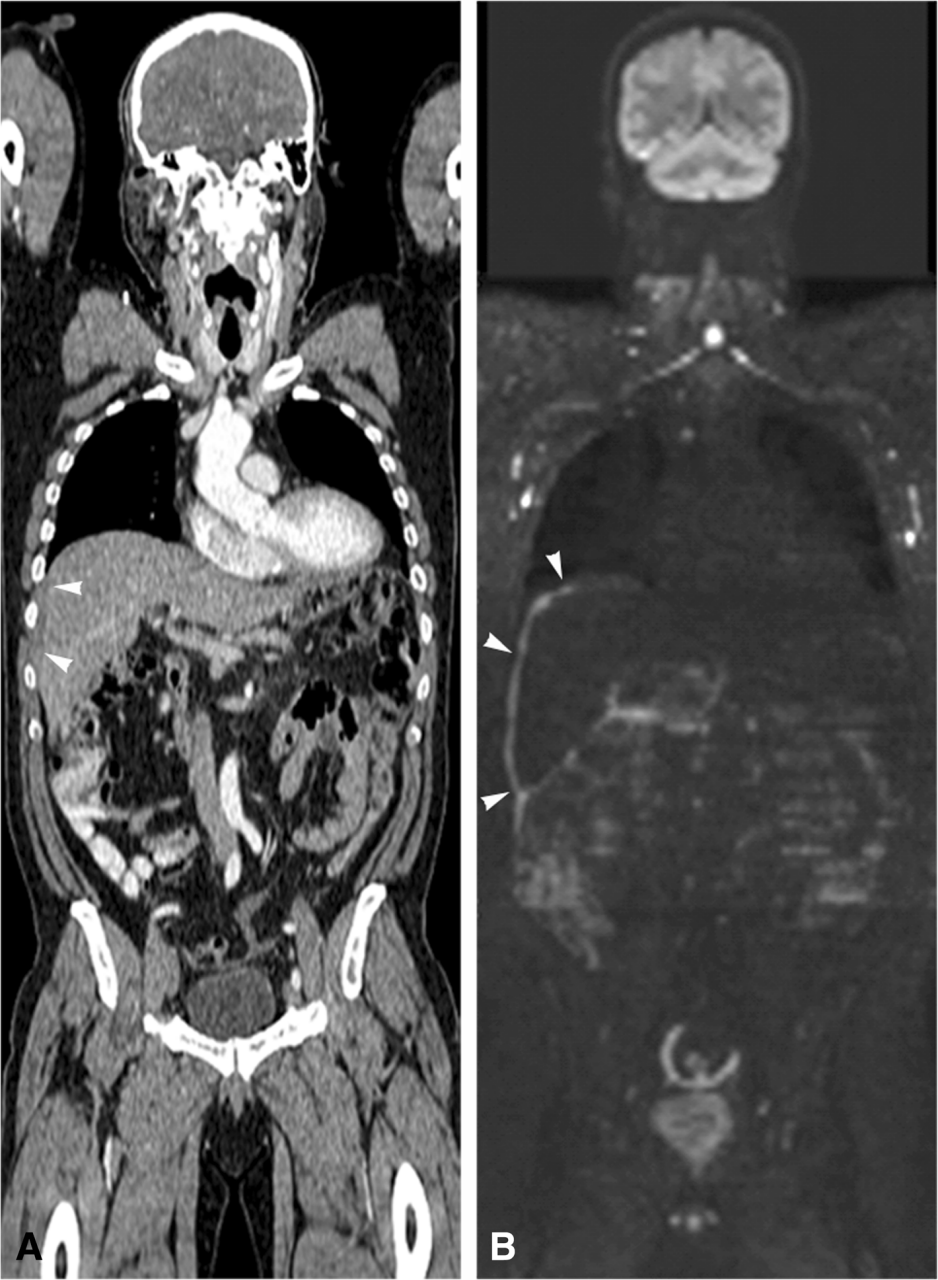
Coronal CT (a) and WB-DWI/MRI b1000 (b) image of a 73 year old male patient with a primary tumour in the descending colon and synchronous peritoneal carcinomatosis. CT images estimated the PCI to be 7 (partially shown with the arrowheads in A as a hypodense thickening on the liver surface) and WB-DWI/MRI estimated a PCI of 19 (partially shown with the arrowheads in B as diffuse confluent metastases at the liver surface). The high PCI of 19 was confirmed during explorative laparotomy. The patient was deemed inoperable and received palliative chemotherapy
